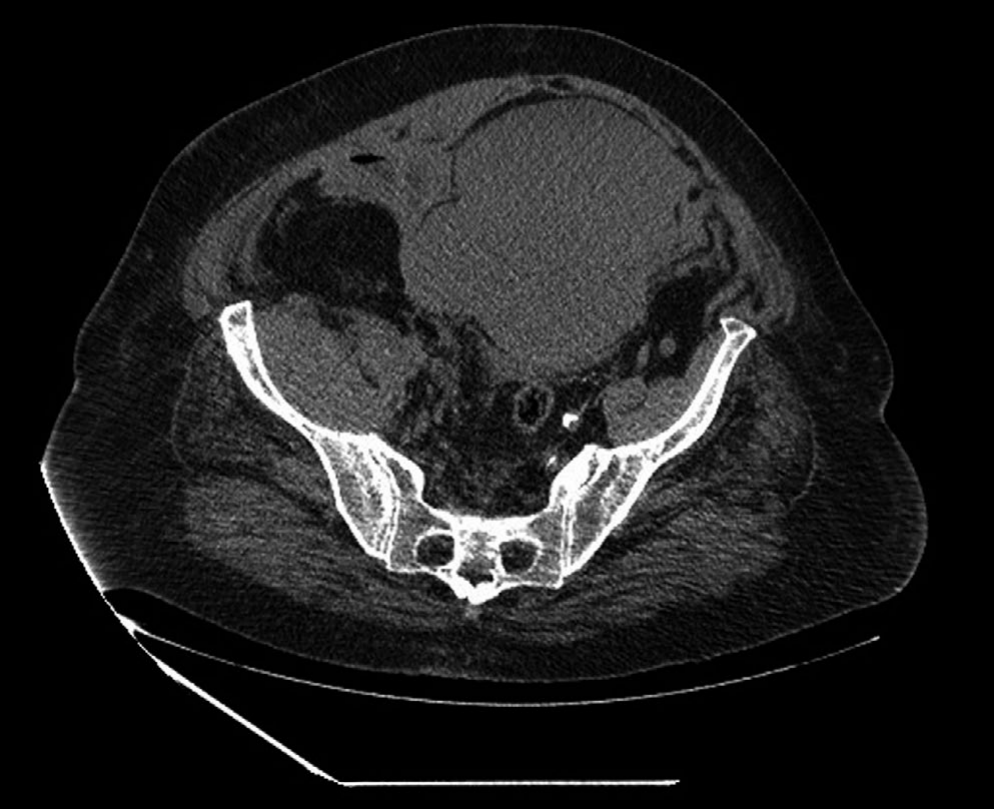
Case 1: CT scan of the abdomen and pelvis revealing a rectus sheath hematoma. Two relatively communicating high‐density expansive formations, the largest, anterior to the bladder, and the other, slightly lower and lateralized to the right, extending to the meso rectum, compatible with two collected hematomas
